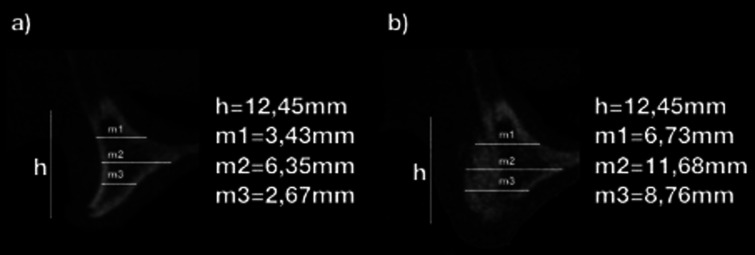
Measures in the CBCT before bone graft (a) and before implant placement (b).
